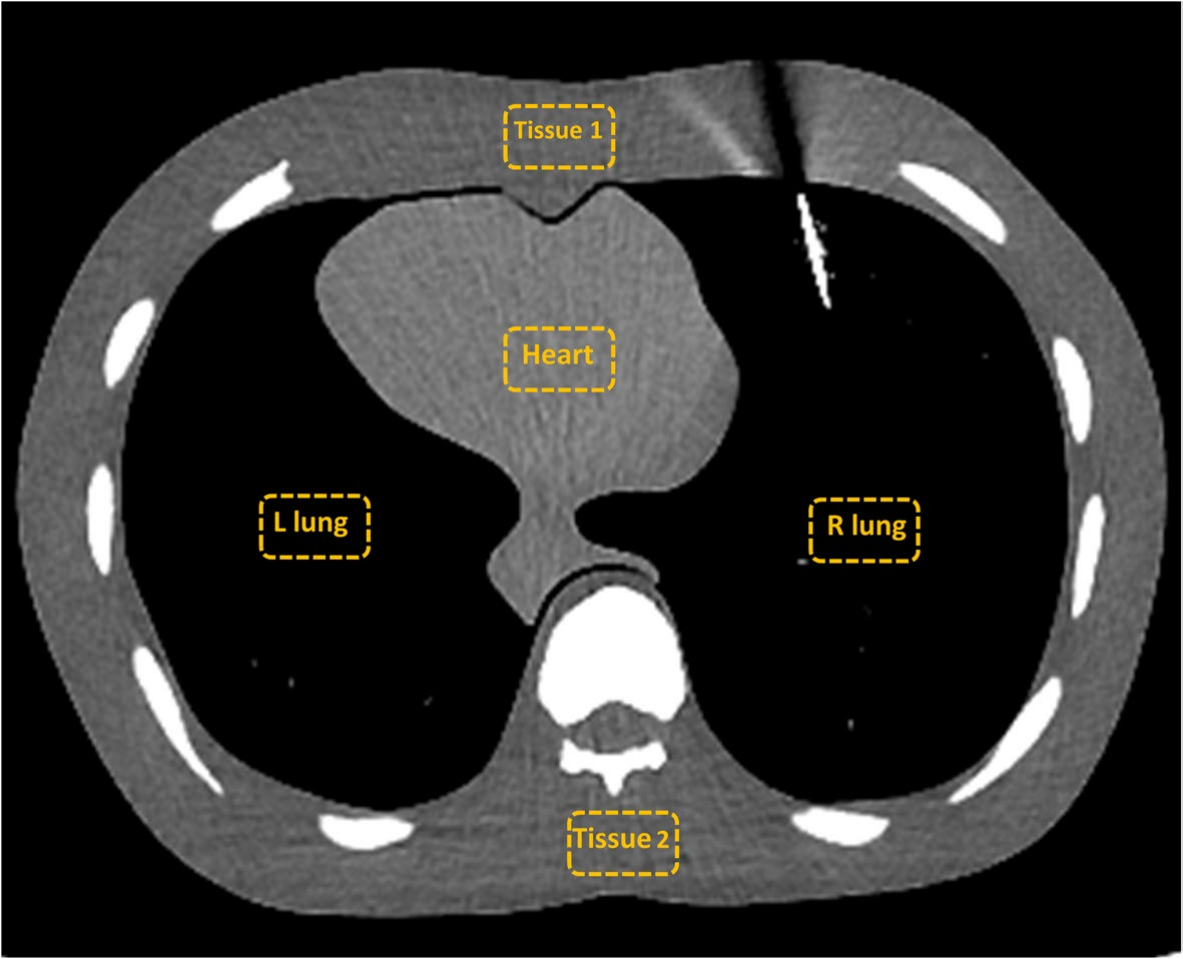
CT image showing the ROIs positions (yellow rectangles) used to measure the image noise
